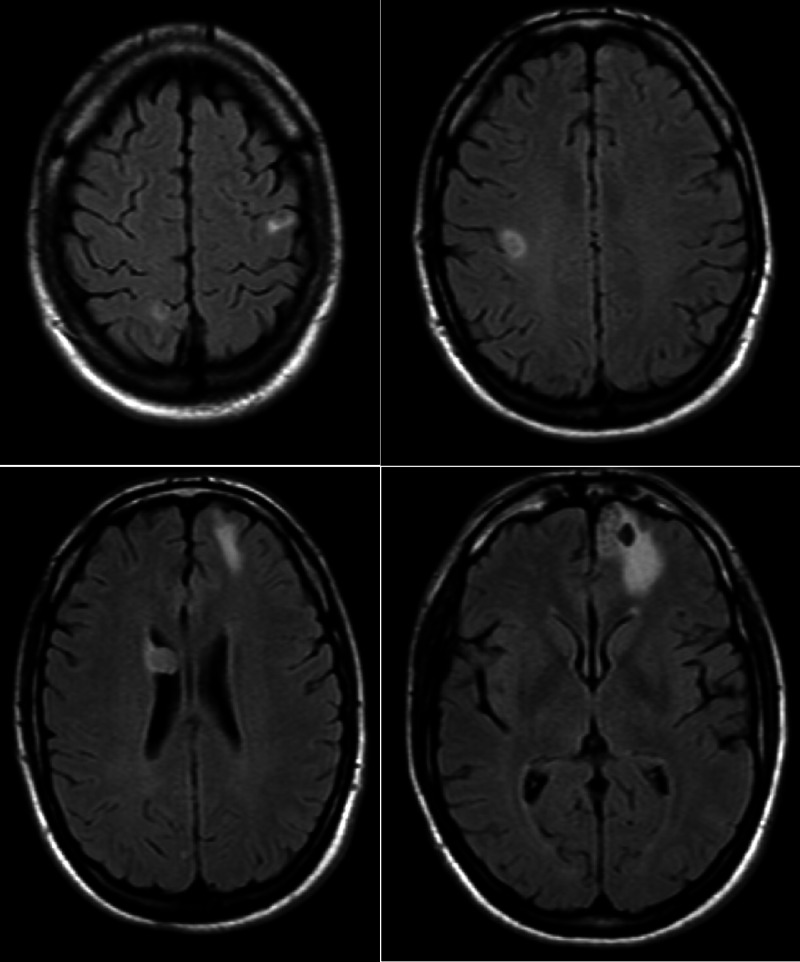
MRI brain with and without contrast showing multiple brain metastases
